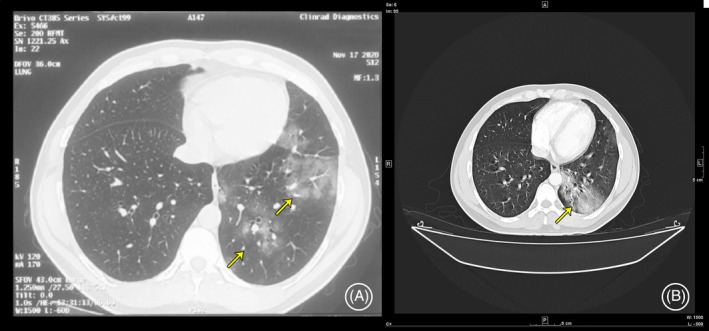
Computed tomography thorax in (A) November 2020 and (B) February 2021 (A, left lower lobe evolving consolidation; B, persistent left lower lobe consolidation)
